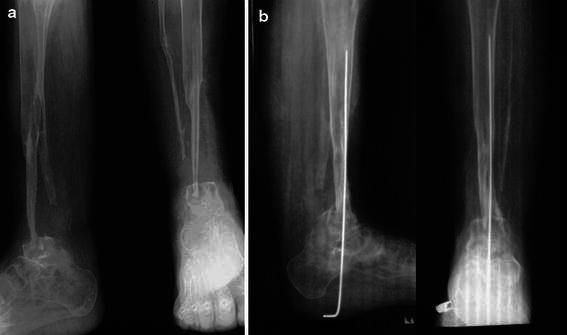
a Radiographs of a 20-year-old male, showing nonunion at the arthrodesis site. A streak of new bone formation from the remnant periostium can be seen at the site from which the fibla was harvested. b Secondary bone grafting was done, along with long K-wire fixation: radiographs at final follow-up showing union at the arthrodesis site along with hypertrophy of the fibula
